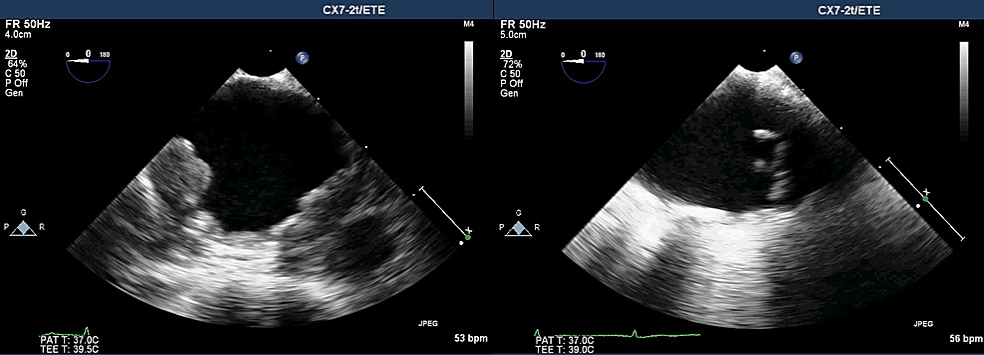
TEE multiple complex atherosclerotic plaques with associated thrombi in the descendent aorta (left) and vegetating mass of erratic movements (7x1.5 mm) in the transition of aortic arch (right). TEE: transesophageal echocardiography
